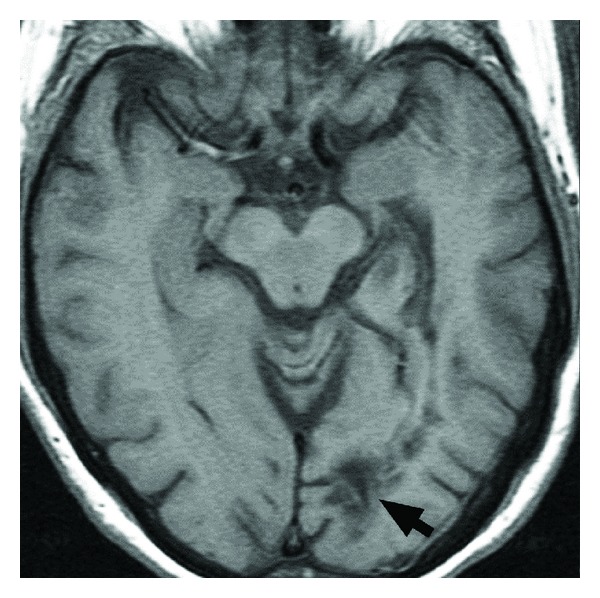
Axial T1 MRI shows evidence of a small left parietooccipital infarction yet the patient was determined to be an unsafe driver.
